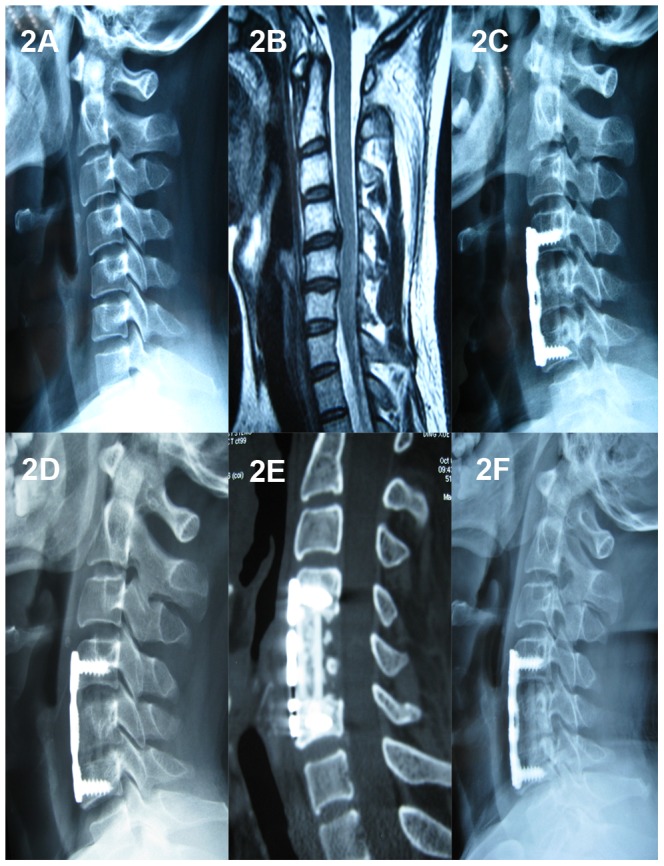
A 36-year-old male who underwent 1-level corpectomy with a nano-hydroxyapatite/polyamide66 cage used for cervical reconstruction. The preoperative cervical X-ray film (2A) and MRI scan (2B) show the spinal cord compression resulting from C4/5 and C5/6 disc herniations. The immediately postoperative lateral X-ray (2C) shows C5 corpectomy and the n-HA/PA66 cage used for reconstruction, and an obvious radiolucent gap can be observed between the cage and the endplates. The lateral X-ray film (2D) shows no obvious radiolucent gap, and the 3D-CT (2E) scan shows the autogenous bone granules filling the cage and achieving bony fusion with adjacent endplates at the 1-year follow-up. A lateral X-ray film (2F) at the final follow-up (four years and eight months) shows satisfying bony fusion and no obvious migration or subsidence.
